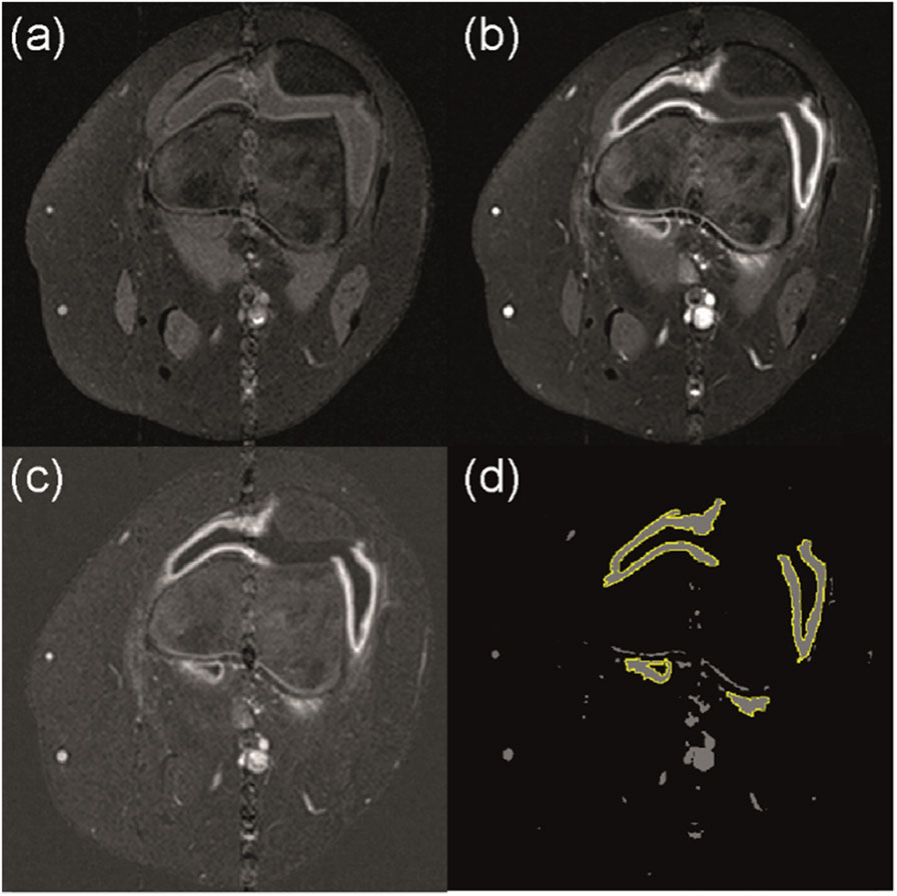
Procedure for calculating the synovial volume, shown on one slice of the imaged knee volume. The acquired data are (a) the multi-slice T1-weighted FS TSE pre-contrast and (b) post-contrast showing synovial hypertrophy. (c) These image stacks are subtracted to produce a stack of difference images which highlight the signal change caused by contrast uptake compared to other tissues; (d) thresholding these values helps to segment the enhancing synovium from structures with low uptake, though blood vessels remain visible. The magic wand tool of ImageJ is used to select the enhancing synovium; the volume is calculated by summing across the image stack. FS TSE, fat saturated turbo spin echo
